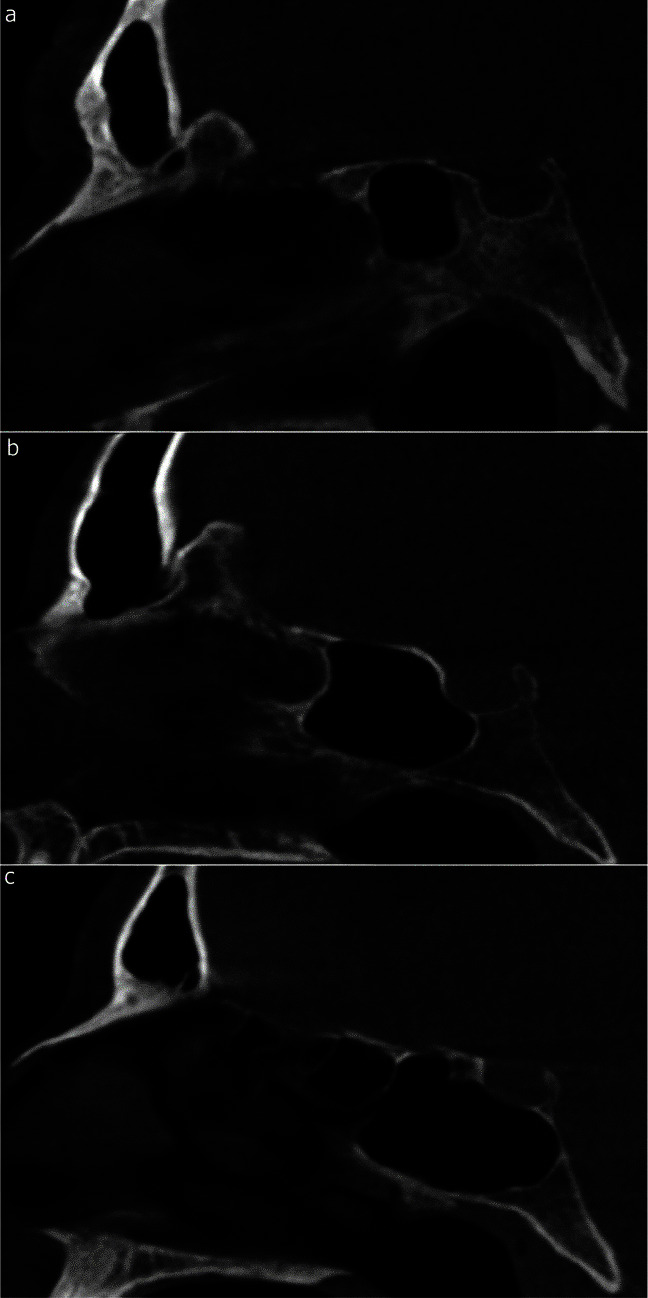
Different types of sphenoid sinus pneumatization. Midsagittal cone beam computed tomography images of sphenoidal sinus morphology; a: Presellar
